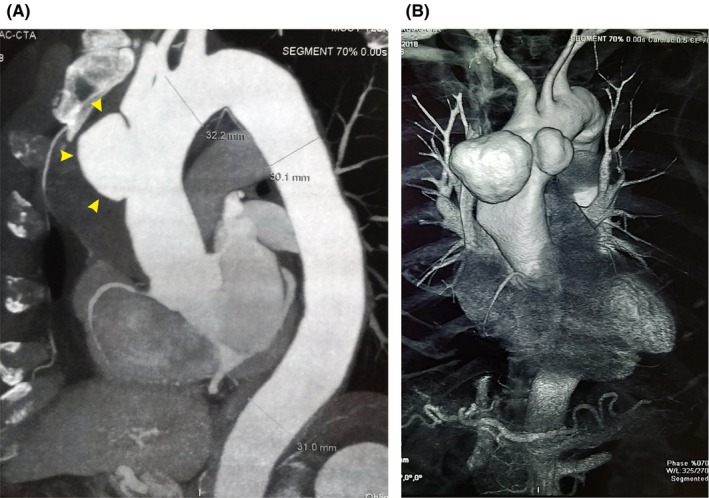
A, Contrast‐enhanced CT scanning and B, CT angiography of the chest in case 2. A mushroom‐shaped aneurysm of the ascending aorta is shown (yellow arrowheads)
